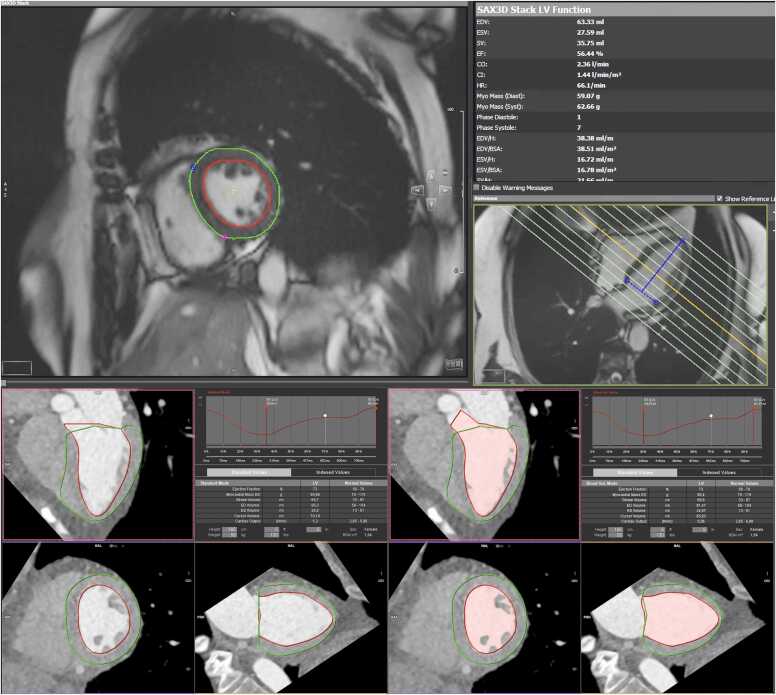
MRI analysis software CVI 42 (top). Bottom left - CT “standard mode” measurement”. Bottom right: CT “Blood Volume” mode, with exclusion of papillary muscles by attenuation thresholding.
